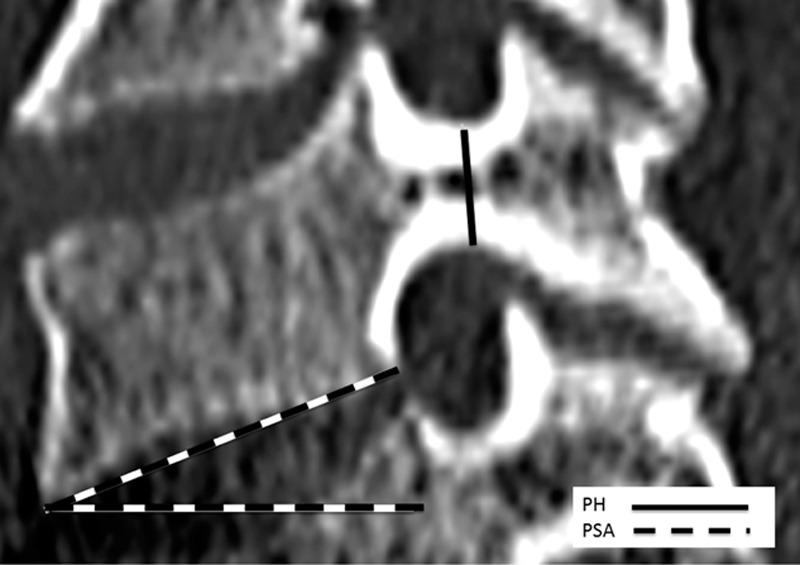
Sagittal computed tomography reconstruction.
